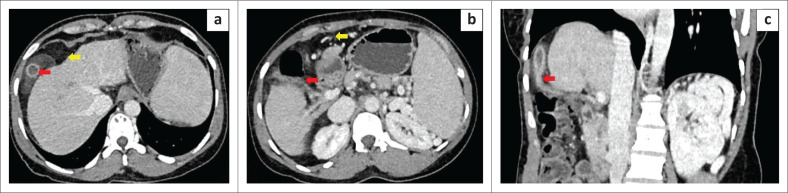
Contrast-enhanced CT images demonstrating a suprahepatic gallbladder in a patient with cirrhosis. (a) Axial image demonstrates an ectopic gallbladder (red arrow) situated along the superior surface of the right hepatic lobe with associated pericholecystic oedema. Cirrhotic liver morphology is evidenced by surface nodularity and irregular hepatic contour (yellow arrow). (b) Axial image at the level of the gallbladder fossa shows continuity of the ectopic gallbladder with the cystic duct (red arrow), while prominent omental collaterals (yellow arrow) indicate portal hypertension. (c) Oblique coronal reformatted image clearly delineates the suprahepatic position of the gallbladder (red arrow), located between the hepatic dome and the right hemidiaphragm.
